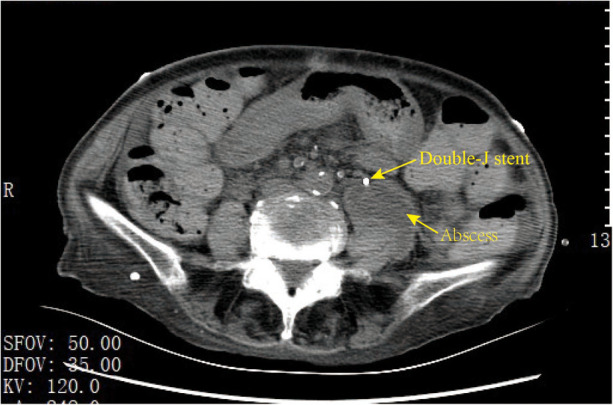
Abdominal CT image on the 12th postoperative day.
